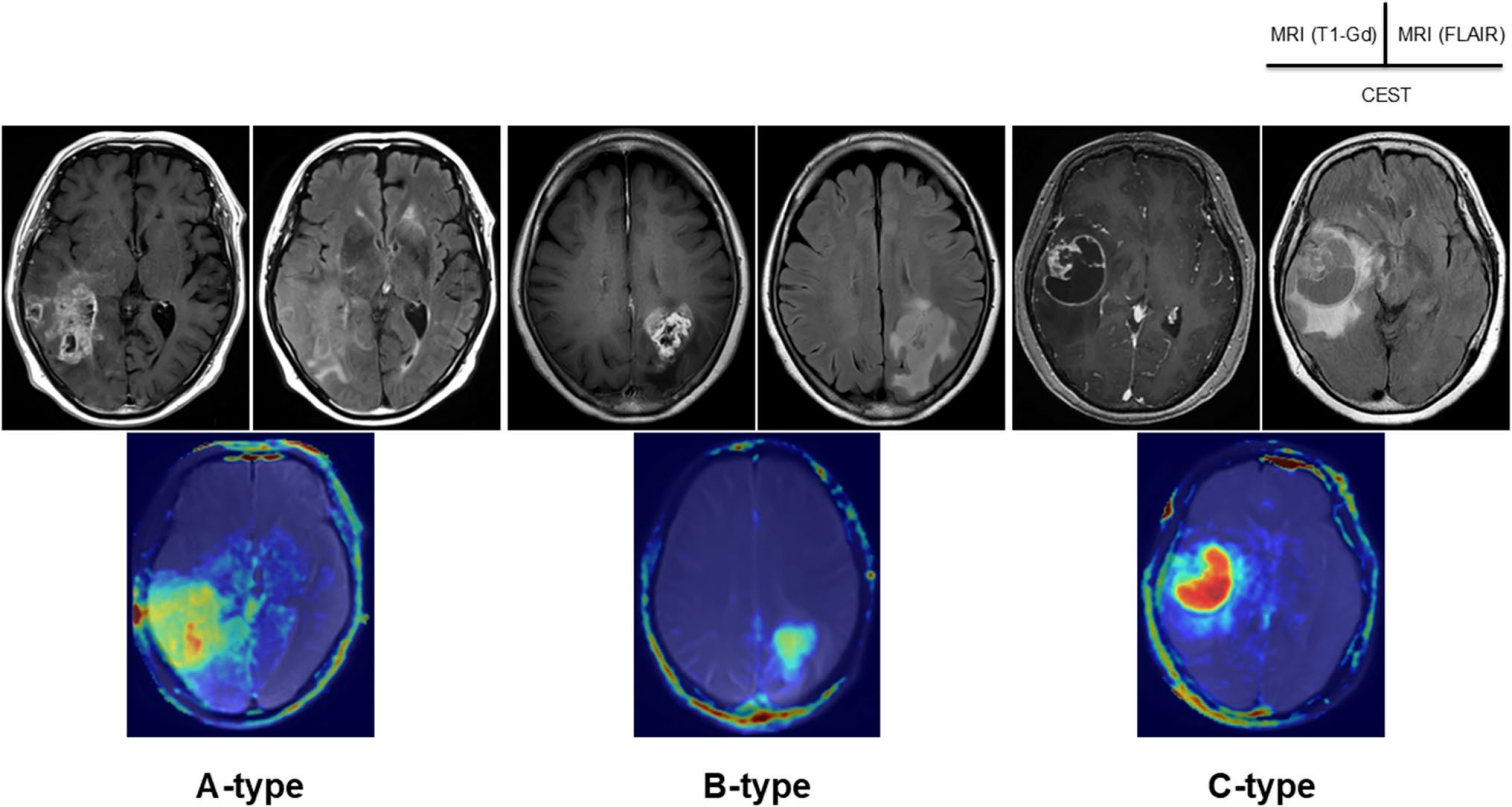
Classification of GBM into three types according to the imaging features of MRI with the assistance of 3D FSE-APT. A-type: high invasiveness with irregular tumor margin and diffuse peritumoral edema. B-type: low invasiveness with relatively well-demarcated tumor margin and focal edema. C-type: high invasiveness with demarcated tumor margin and diffuse edema. All three types showed signal hyperintensity from APT on 3D FSE-APT
